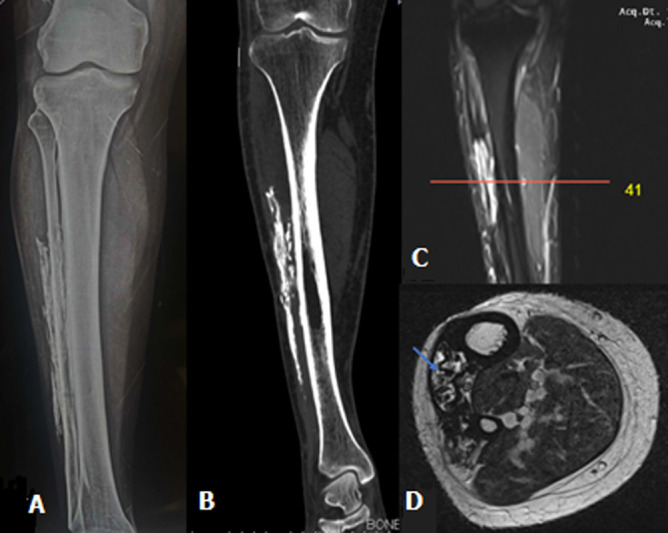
A) X-ray of the right leg demonstrating linear, sheet like, eggshell calcification in the soft tissue compartment laterally adjacent to the fibula in the mid-leg region showing no evidence of bone involvement or periosteal reaction; B) CT of the right leg coronal view, demonstrating peripheral sheet-like calcification along the long axis of the limb within a fusiform intramuscular lesion; C,D) MRI of the right leg (coronal and axial views) showing a fusiform intramuscular lesion with a low-signal peripheral rim and central T2 hyperintense calcified content (blue arrow)
